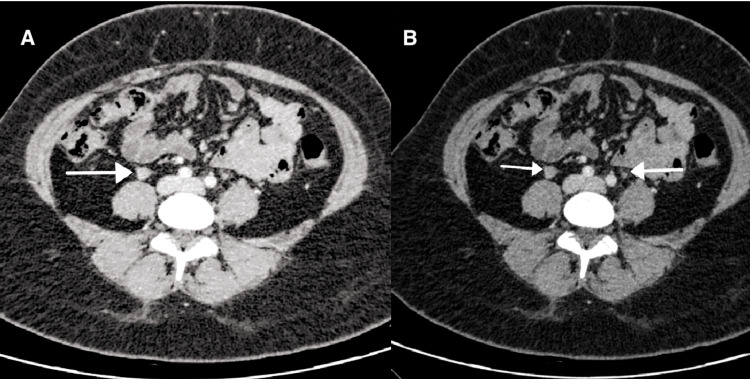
(A) CT scan (axial section, venous phase) showing dilated right ovarian vein anterior to the psoas muscle with subtle stranding (arrow). (B) CT scan (axial section) showing thickened right gonadal vein compared to the left (arrows). CT: computed tomography
